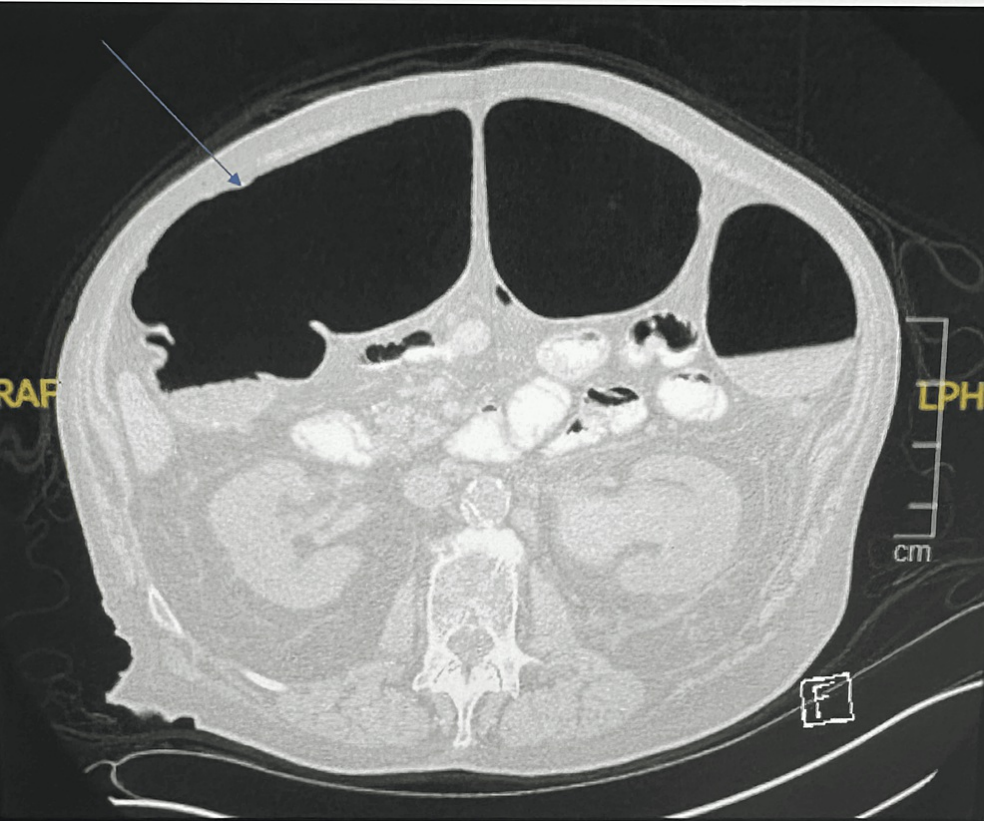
CT imaging of the abdomen and pelvis shows diffuse dilatation of the proximal colon.
